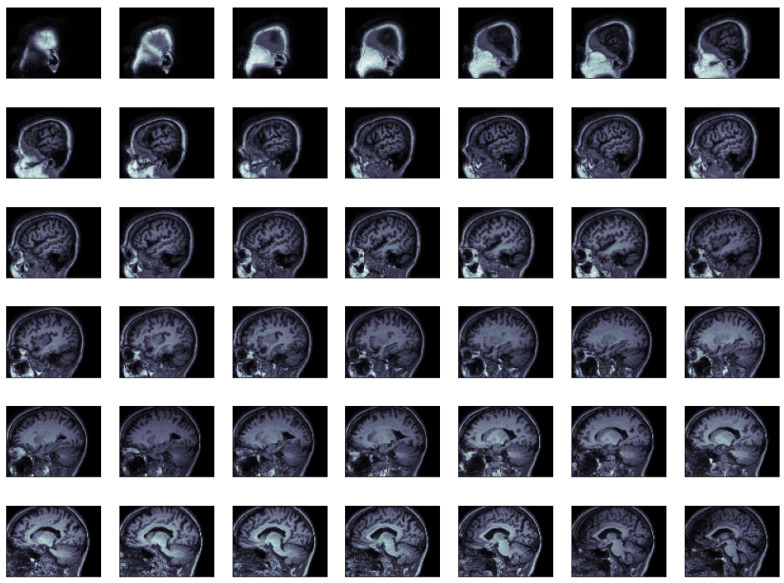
Processed volumetric data from a subject’s MRI (extract of the processed slices).
